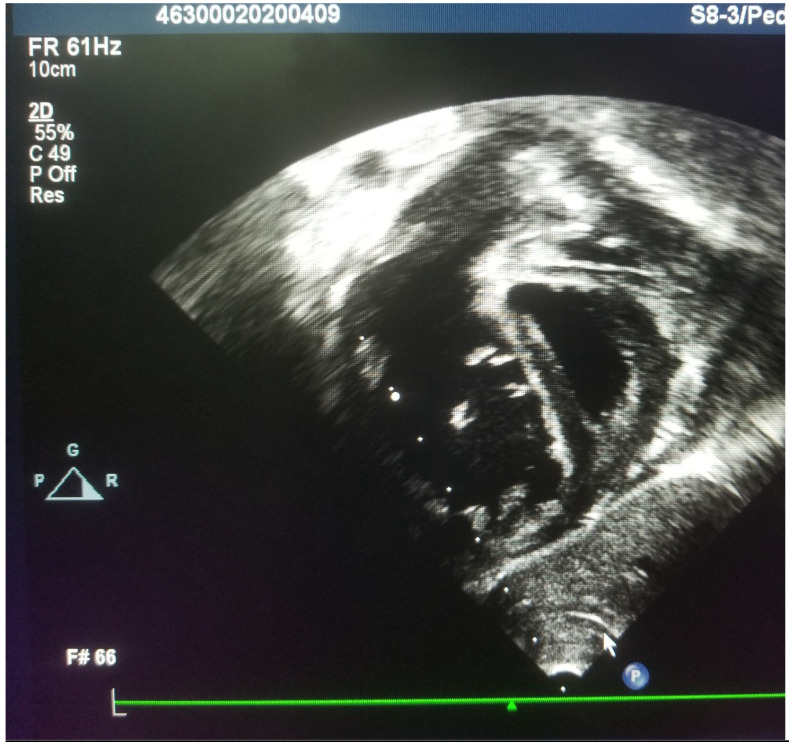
Still frame echocardiography image of subcostal short axis view at the right ventricular outflow tract displaying flat interventricular septum and D- shaped left ventricle suggesting severe pulmonary hypertension
